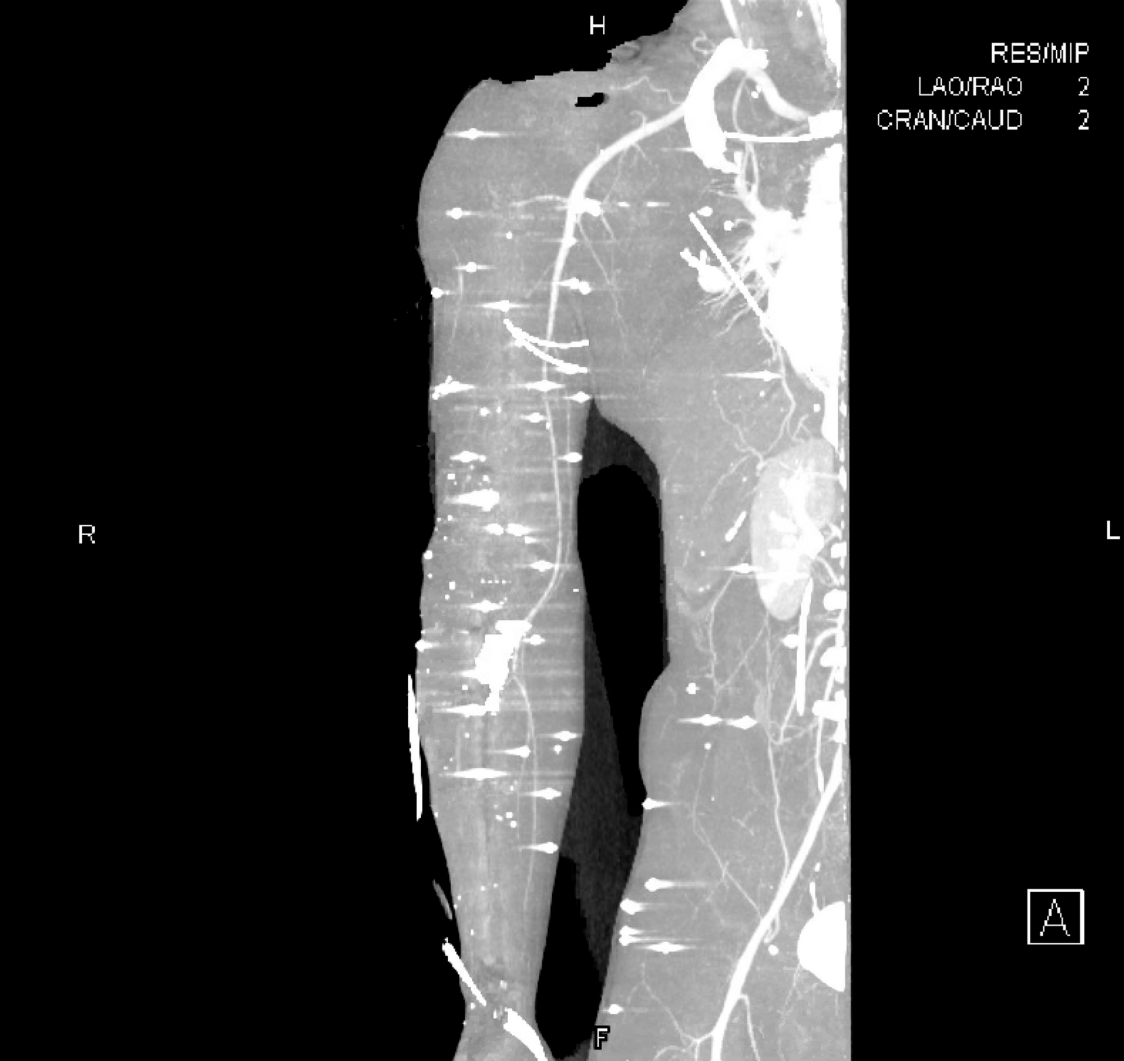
CTA MIP right upper extremity Image shows pellets with streak artifacts of the right upper extremity and right abdomen
